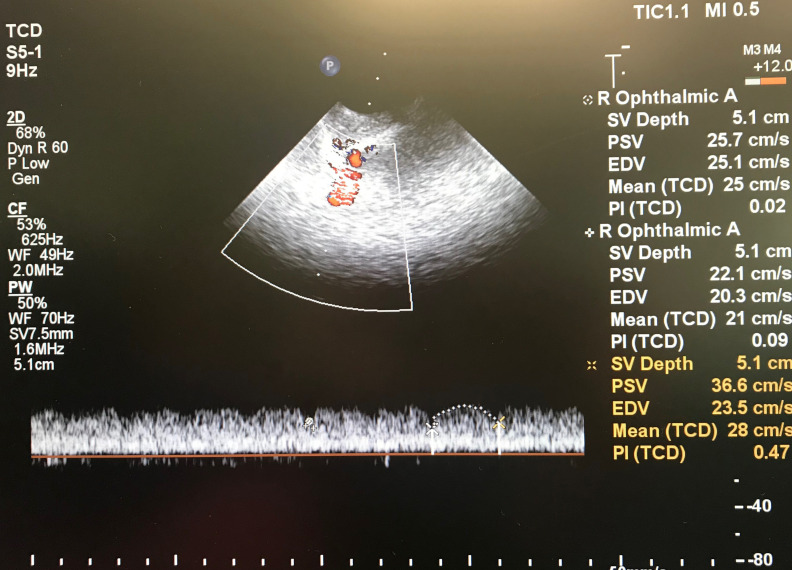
Colour Doppler imaging of the ophthalmic artery, at lowest temperature of cardiopulmonary bypass (28°C). OA mean velocity: 28 cm sec^−1^.
